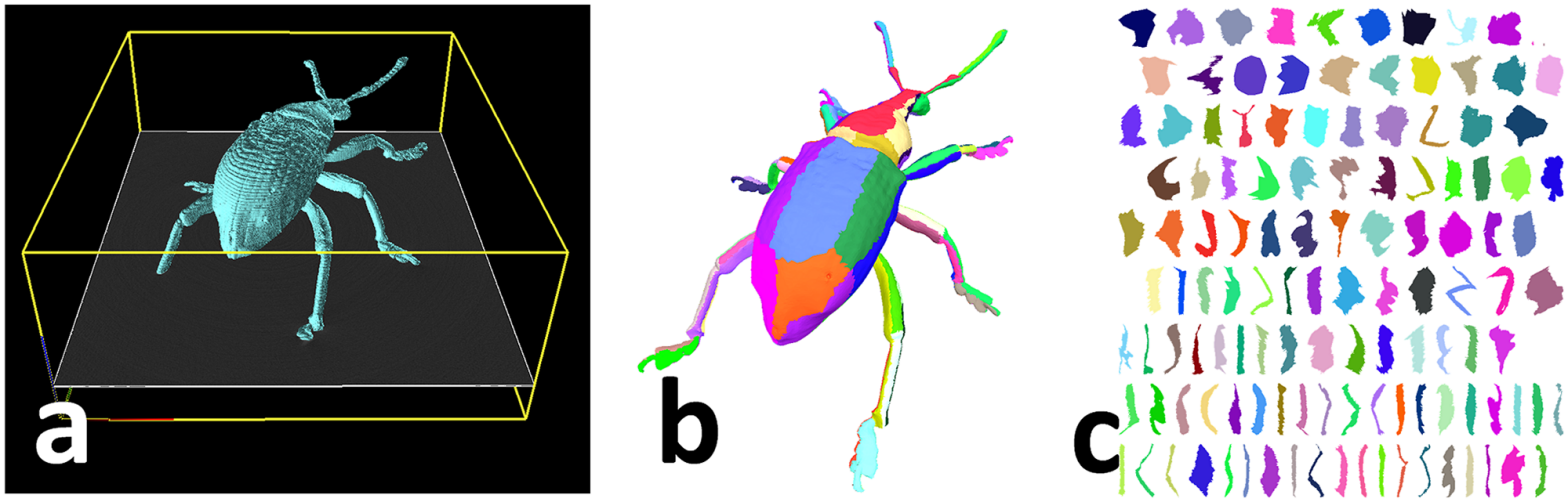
Surface reconstruction. We extracted foreground from input CT volume (a) to obtain surface model (b). We segmented surface into near-flat regions (b) and flattened each region into 2D texture domain (c).
